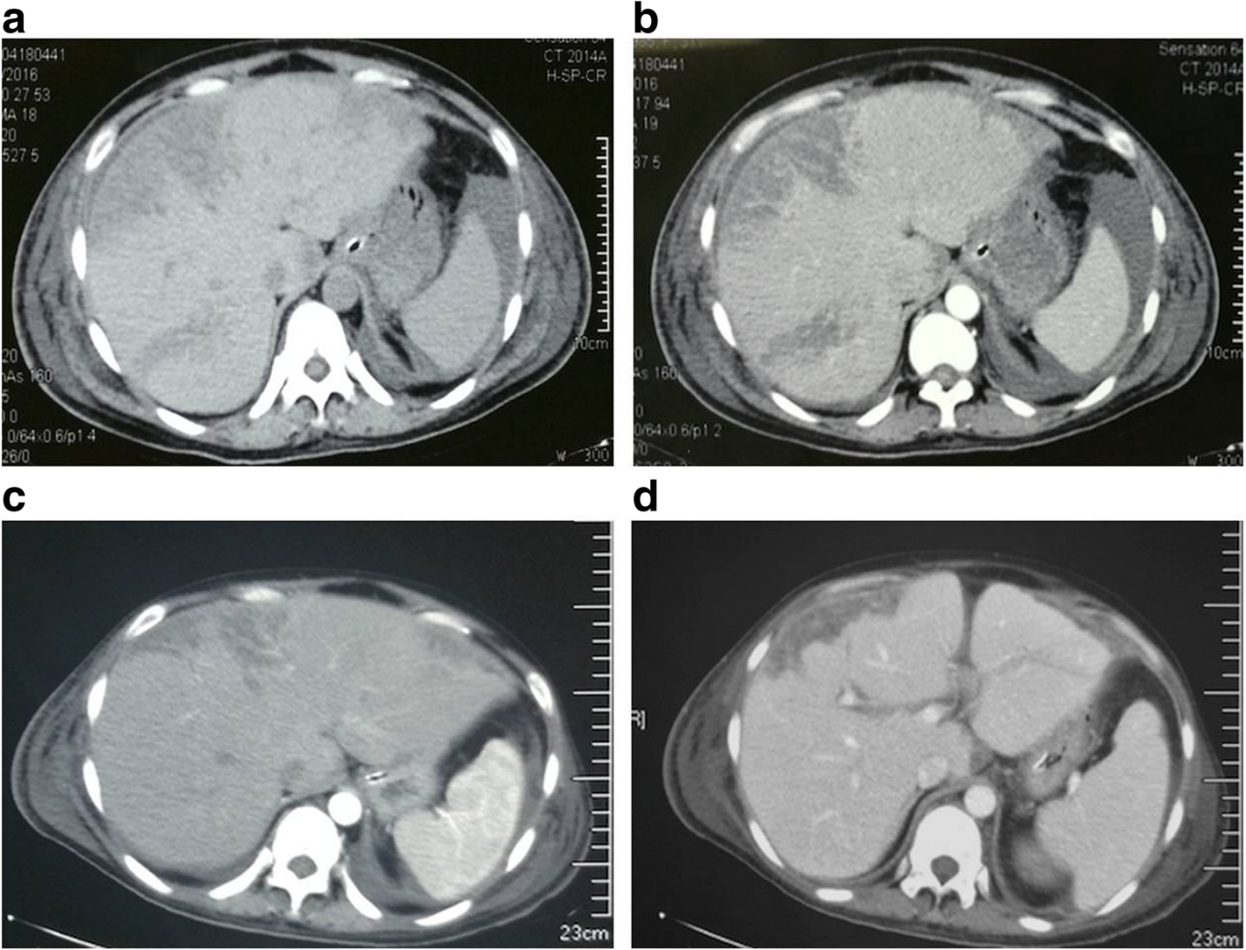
a Non-contras CT demonstrates peripheral wedge-shaped inhomogeneous low attenuation in the right hepatic lobes on 3th after admission. b Contrast-enhanced CT of the liver shows mottled appearance in the same areas where vessels were seen coursing through. c and d showed the damaged areas were reduced in the enhanced CT 17th days after admission
